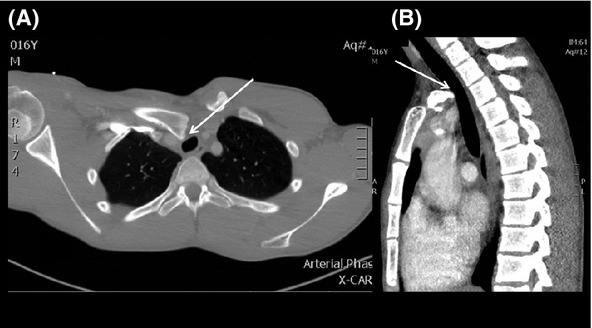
Multi-slice computed tomography demonstrating posterior dislocation of the sterno-clavicular joint and abutment and displacement of the trachea. These pre-operative images, (A) axial and (B) sagittal, demonstrate how the medial end of the clavicle is dislocated and is abutting and compressing the trachea (arrow).
